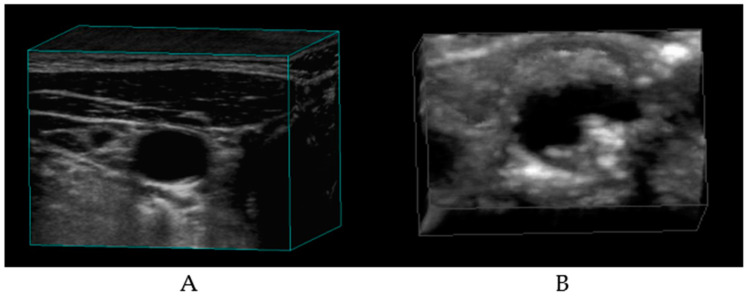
Three-dimensional ultrasound images of carotid arteries. (Courtesy: Dr Aaron Fenster, Research Director, Robarts Research Institute, Canada.) (A) Carotid artery with minimal stenosis. (B) Carotid artery with plaque.
